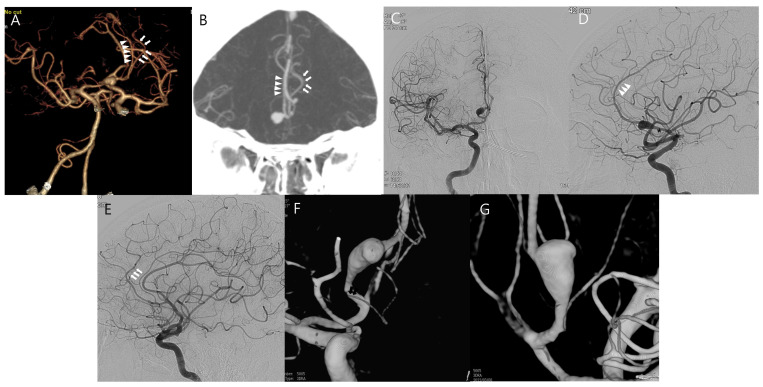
Brain images from a 65-year-old male who presented with sustained headache. (A,B) Initial computed tomography angiography shows a fusiform aneurysm at the A2 segment of the anterior cerebral artery. (C,D) Right antero-posterior and lateral internal carotid artery angiogram shows fusiform aneurysm at A2. Note that the proximal portion of the aneurysm (asterisk) is deep and anteriorly deformed parallel to the aneurysm. (E) The caliber of left A3 (white arrows) is similar to right A3 (white arrowheads) of the bypass site; however, (A,B) there exists significant distance between them. (F,G) The aneurysm is located just distally to the frontopolar artery (black arrows) with bleb and a maximal size of 10 cm.
